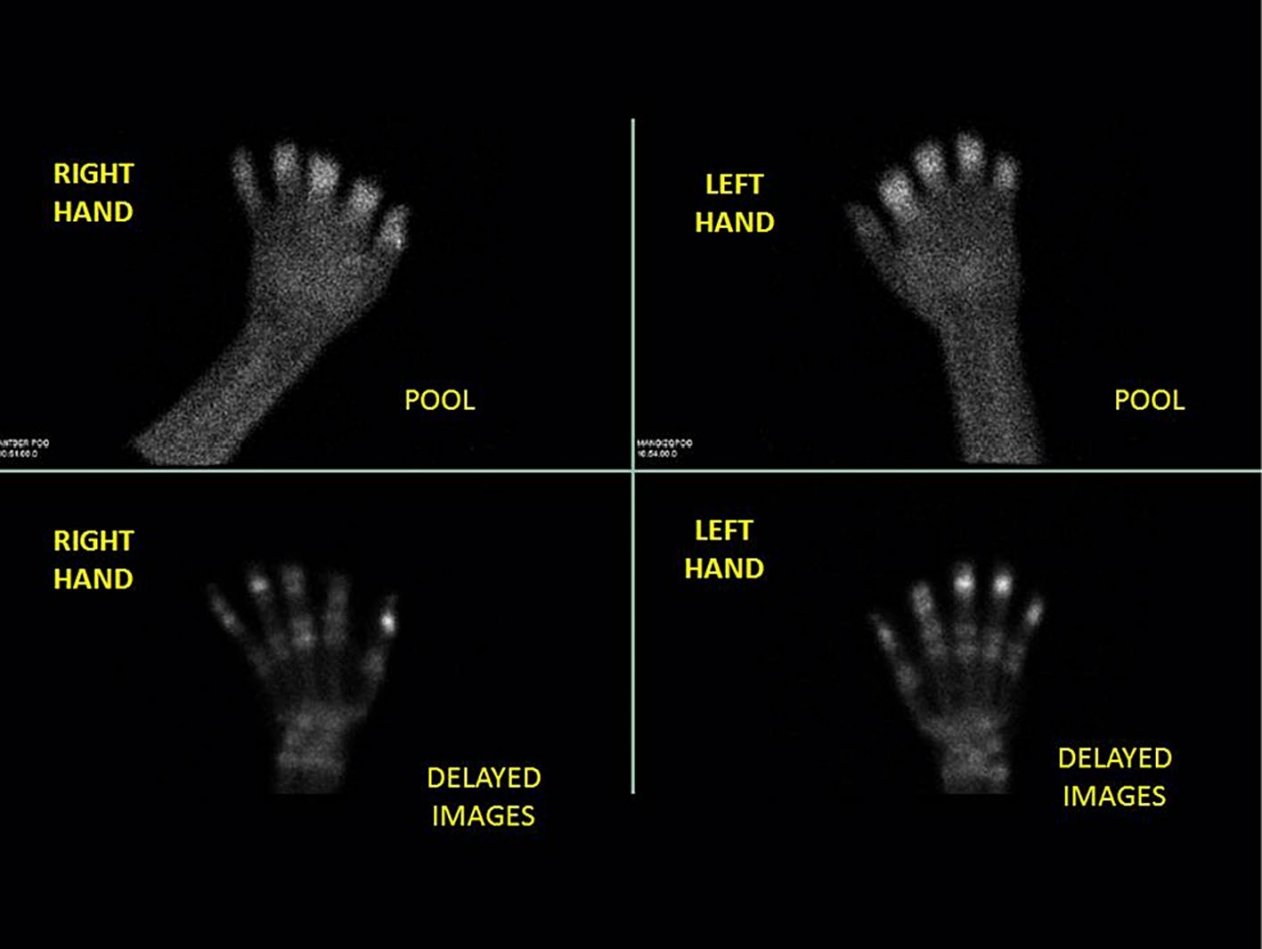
Multiphase 99mTc-MDP bone scintigraphic. Multiphase 99mTc-MDP bone scintigraphic images of the hands show palmar soft tissue phase (above) and 4-h delayed phase (below)
